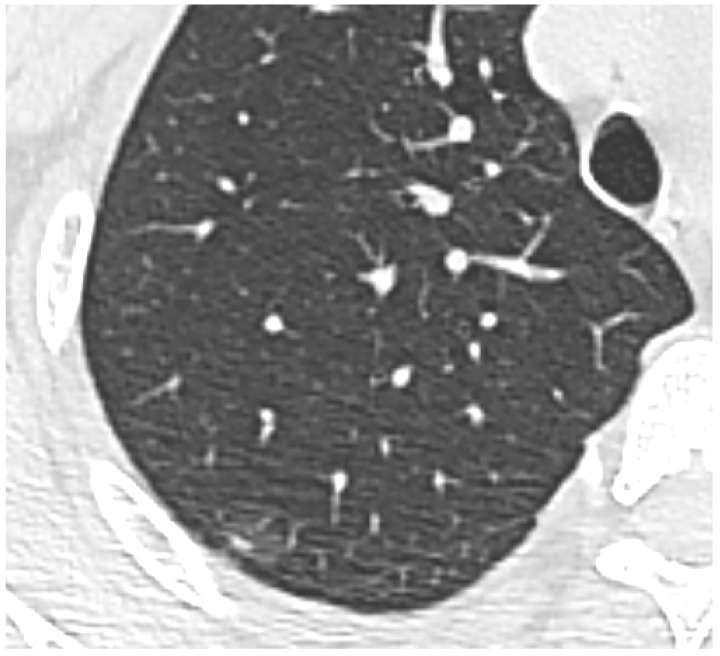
Example of segmentation failure. Magnified HRCT demonstrating a sub-solid nodule in the posterior segment of the right upper lobe. Its irregular margin and immediate proximity to the lung tissue edge (pleura) likely caused the CAD lung segmentation algorithm to exclude the lesion as part of the chest wall.
